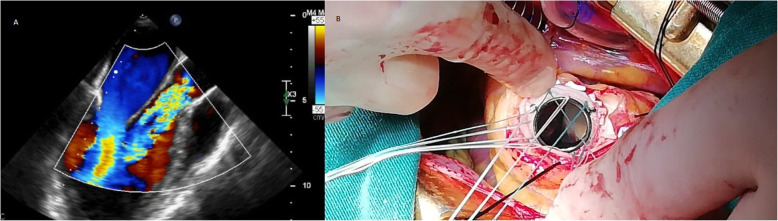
a Transthoracic echocardiogram revealing a maximum aortic velocity of 5.36 m/s. b A 17-mm prosthetic aortic valve is placed
